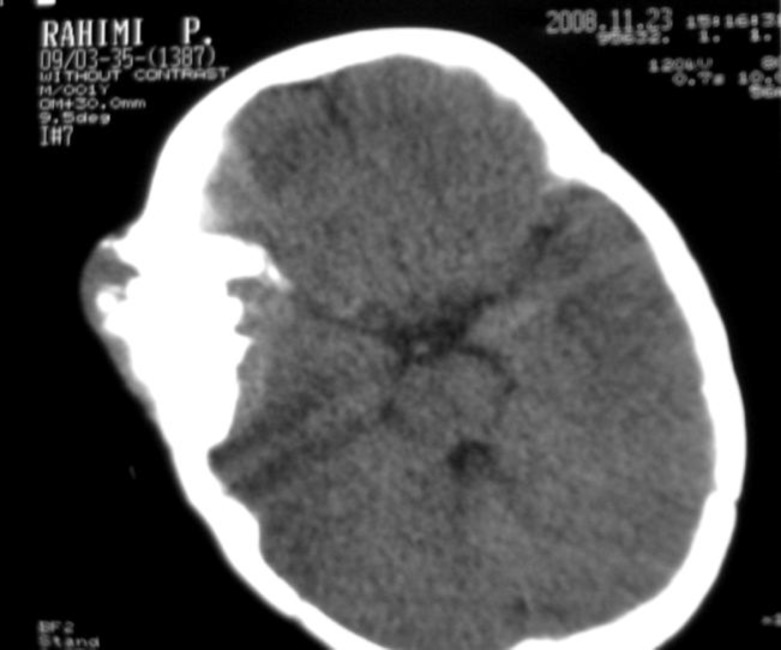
CT scans revealed an intracranial, well-defined, isodense mass, surrounded by a hypodense area and hyperostosis in the right temporal region adjacent to the tumor. Part of the tumor grew extracranially. The tumor was homogeneously enhanced by intravenous infusion of contrast medium, also brain Parenchyma was intact
